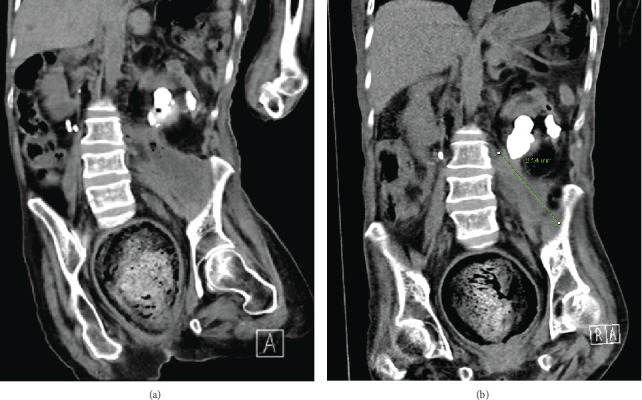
(a, b) CT scan revealing a large left-sided psoas abscess, associated with gas bubbles, observed during the patient's representation several months after initial discharge.
